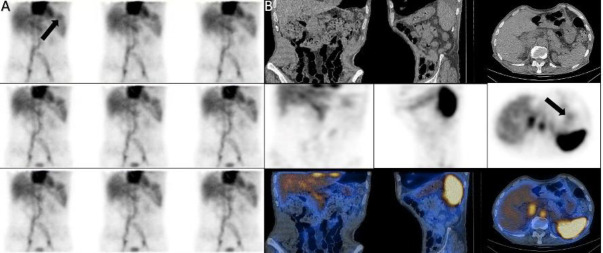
Dynamic (A) and SPECT/CT (B) showed the source of bleeding in stomach (arrows)
